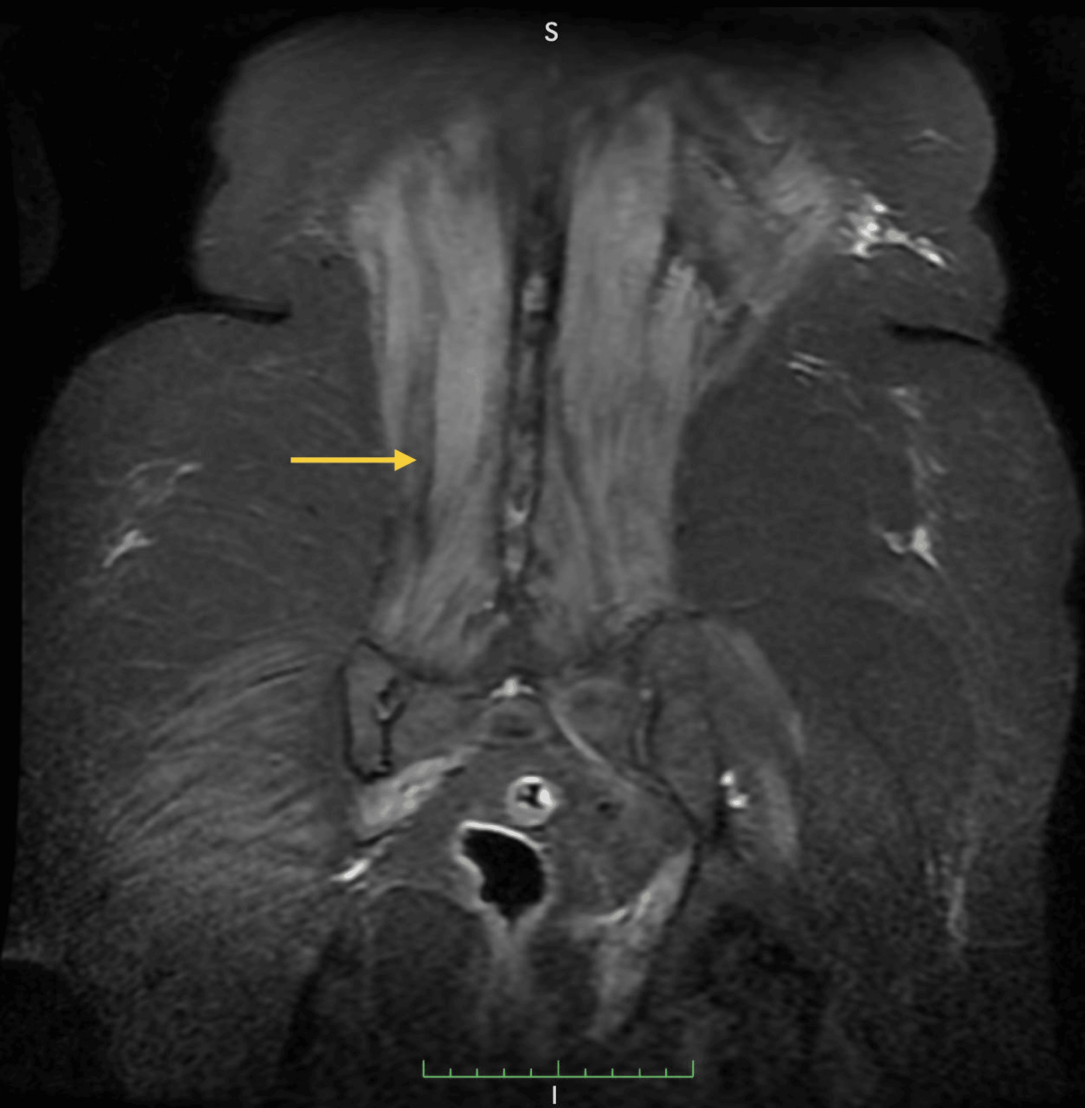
MRI of the spine showing myoedema in paraspinal, piriformis, and obturator muscles The yellow arrow in the figure points toward the myoedema in the paraspinal muscles MRI, magnetic resonance imaging
